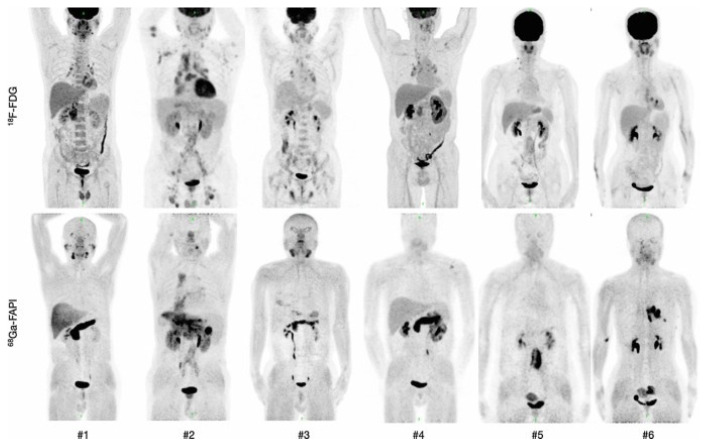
Comparison of FAPI PET/CT and FDG PET/CT in six patients with IgG4-related disease. The FAPI images (lower panels) demonstrate clear uptake in fibrotic and inflammatory lesions—pancreas 1,2,3,4), bile duct/liver (1,2,3), retroperitoneum (5), lung/pleura (6), and salivary glands (1.3)—often exceeding FDG detection sensitivity. From Luo et al. [187].
